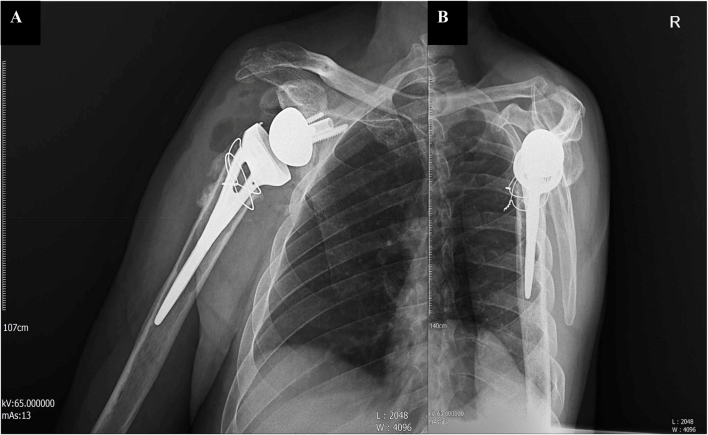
Postoperative anteroposterior (A) and Y-view (B) radiographs showing the reverse shoulder arthroplasty (RSA) in place.
